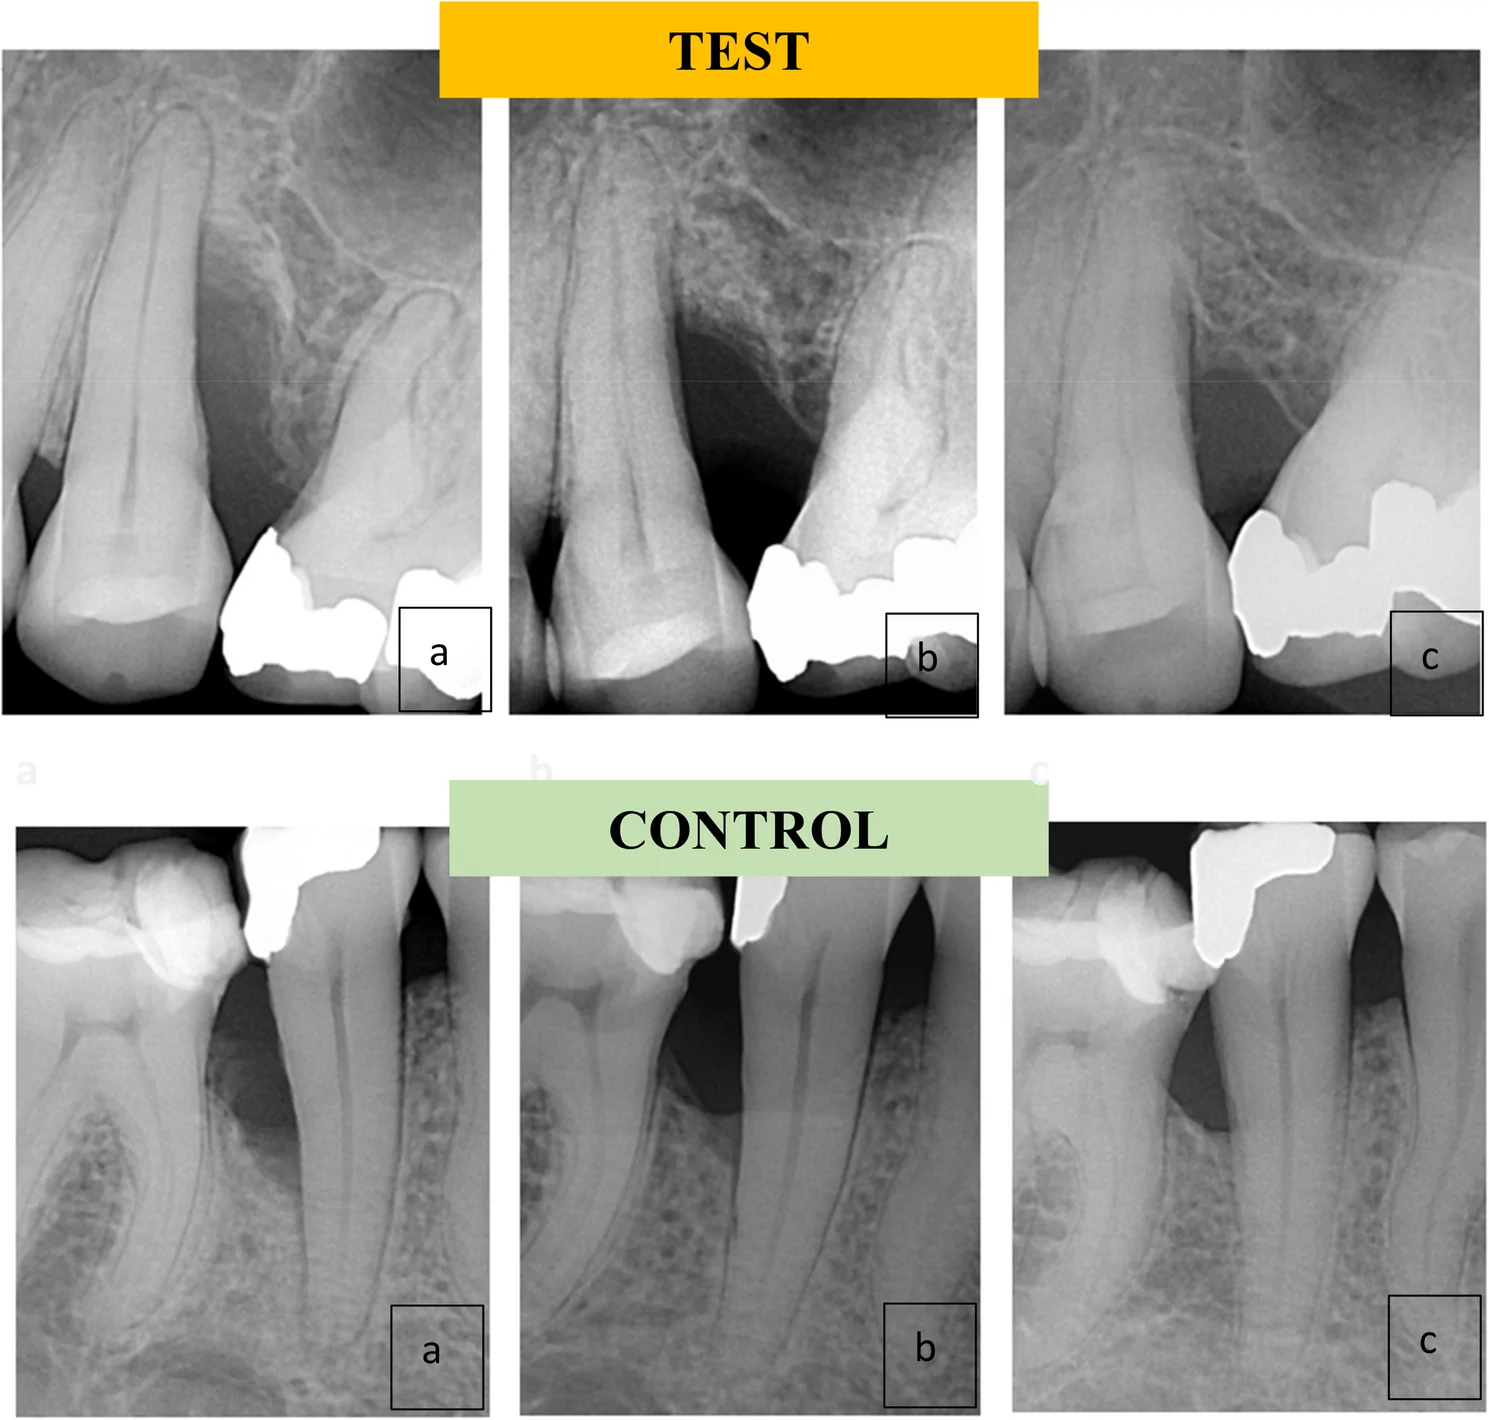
Representative radiographic images of PRF- and EMD-treated intrabony defects obtained preoperatively, at 6 months, and at the 3-year follow-up: (a) preop; (b) 6 months postoperatively; (c) three years postoperatively
